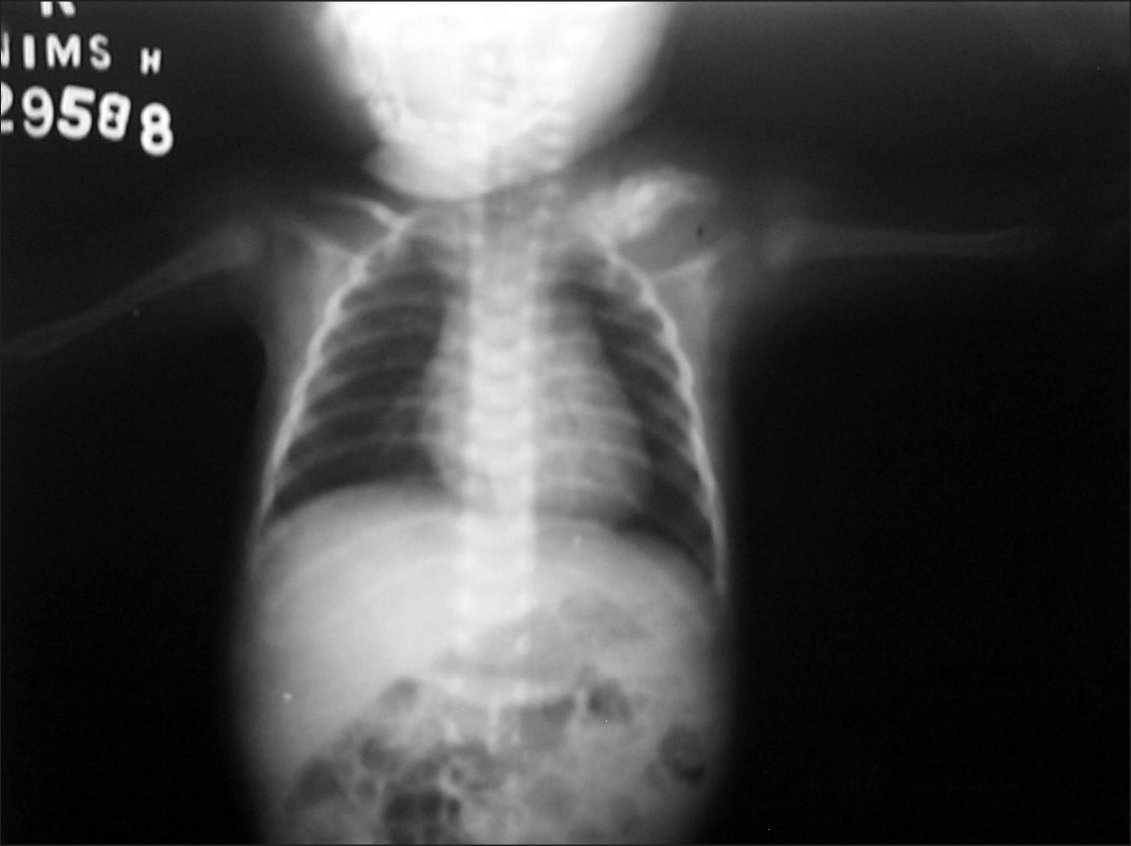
X-ray chest showing bilateral clavicular thickening, sclerosis and overlying soft tissue swelling
